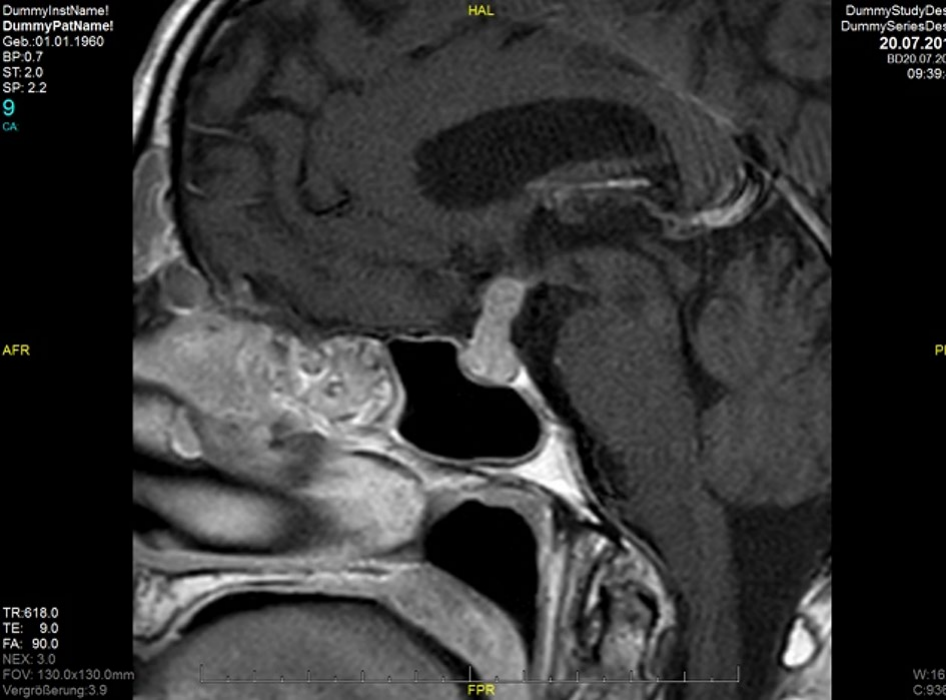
MRI image of typical lymphocytic hypopyhsitis with stalk involvement
